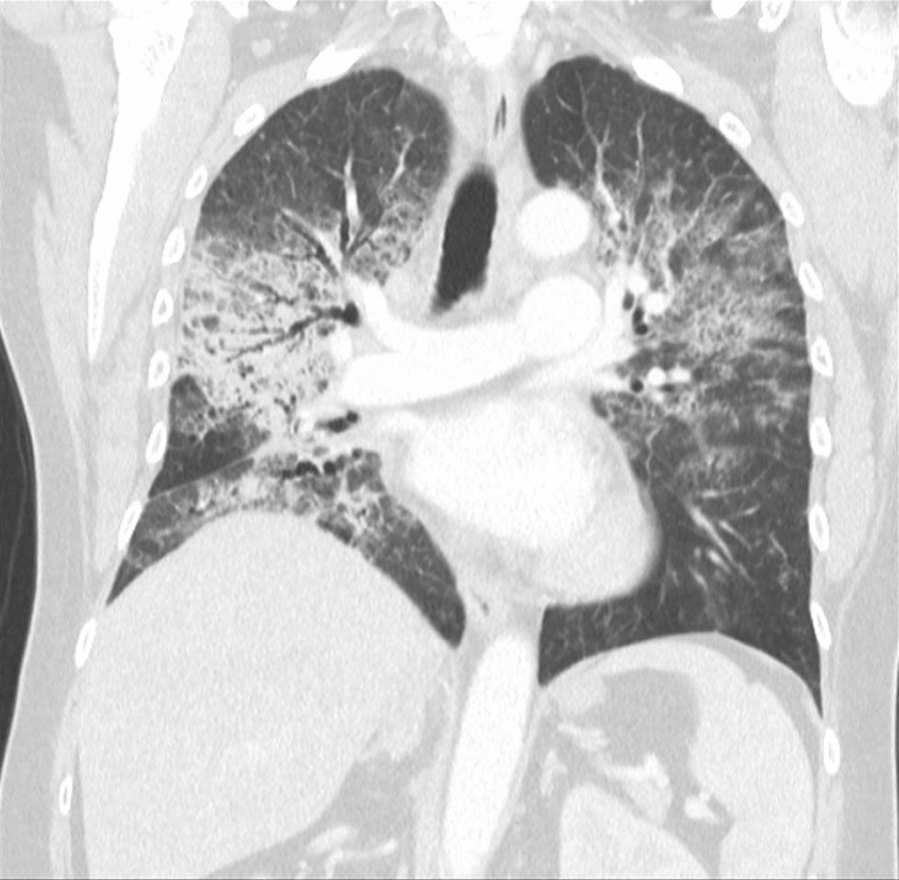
Case 1—chest computed tomography (CT) revealing signs of pneumonitis
